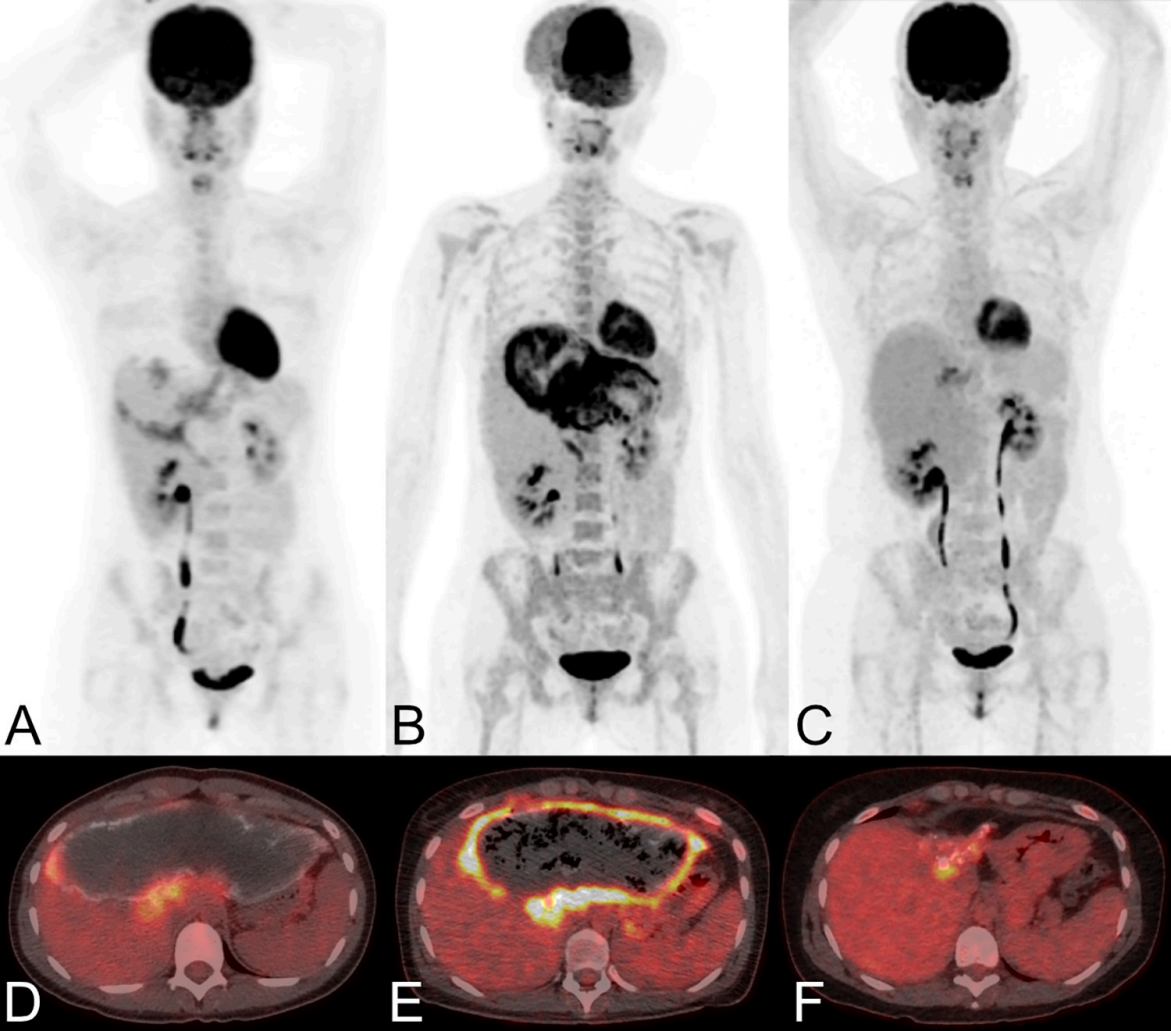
Three PET/CT scans performed in a female patient with a large hepatic manifestation of alveolar echinococcosis. The patient (patient 04 in Table 2) was 24 years old in 2005 (maximum intensity reconstructions of PET (A) and fused PET/CT images (D)), 32 years old in 2012 (B and E), and 40 years old in 2020 (C and F). She was treated with albendazol since 1997 and therapy was ongoing at the last clinical follow-up in 2021. In 2005, PET/CT showed moderate metabolic activity (A and D), EgHF AU were 58. In 2012, the patient presented with fever and was diagnosed with cholangitis and subsequently treated with antibiotics—PET/CT showed intense metabolic activity in the periphery of the echinococcosis manifestation (B and E), EgHF AU were lower than in 2005 (i.e., 46). In 2020 (C and F), PET/CT again showed moderate metabolic activity to a lesser extent, EgHF AU were 13. The large discrepancy in trends in this case, may indicate, that metabolic activity and EgHF antibody levels may have to be evaluated independently, when treating patients with alveolar echinococcosis.
